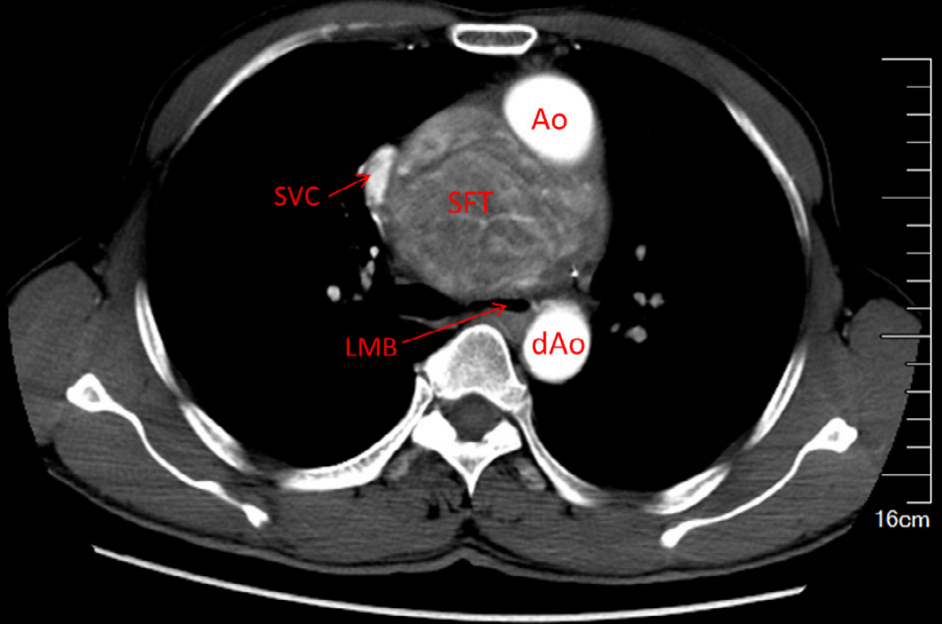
Thoracic computed tomography (CT) scan showing a giant mediastinal mass infiltrating adjacent vital structures.
